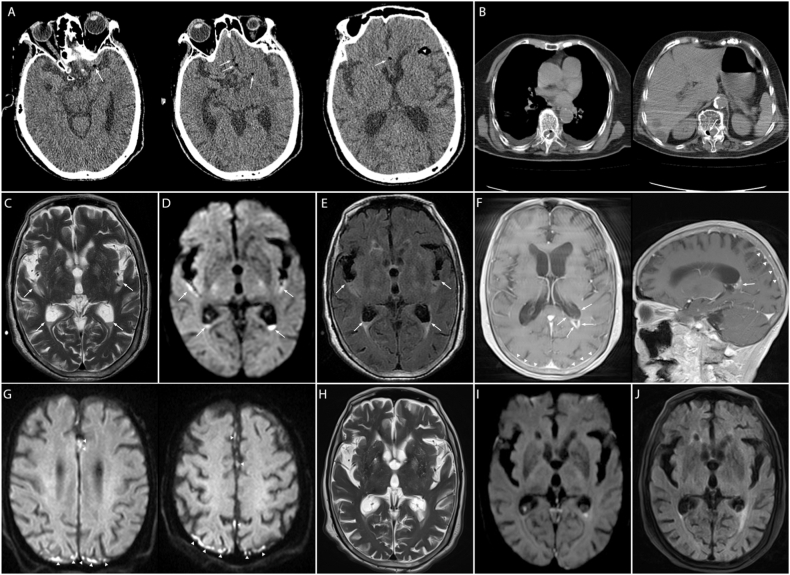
Brain computed tomography (CT) scan, showing pneumocephalus in the subarachnoid space (A). Gas density is present in the spinal canal on chest CT (B). Magnetic resonance imaging (MRI) findings, indicating empyema in the trigone of the lateral ventricle, the Sylvian fissure and the cerebral hemispheric sulcus (C–G), and significantly improved neuroimage changes after treatment (H–J).
